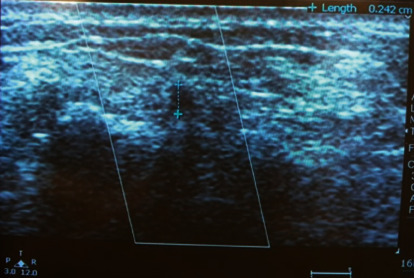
Ultrasound guided left distal radial arterial diameter measurement.
